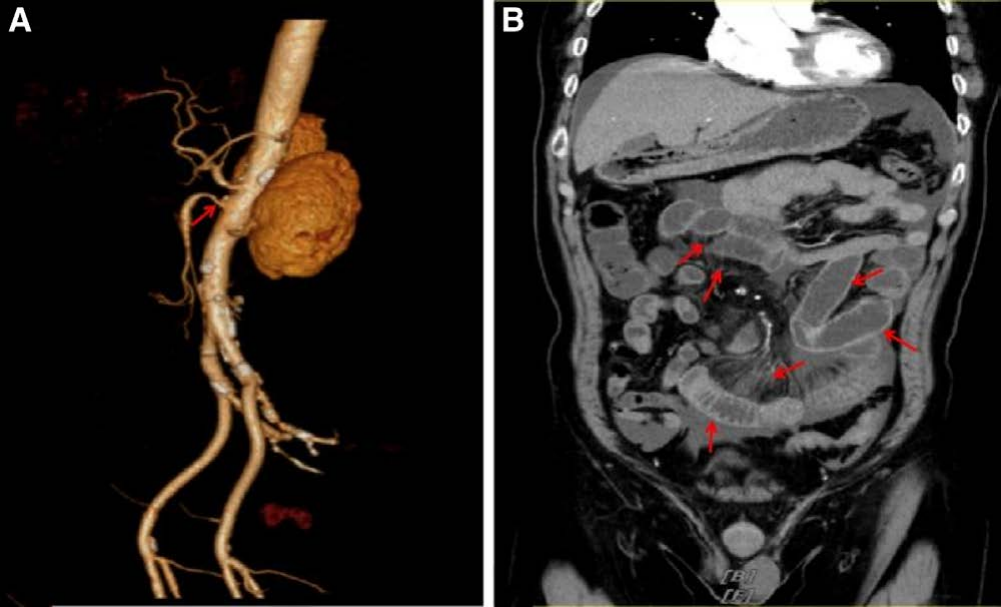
Abdominal CTA examination showing (A) stenosis at the opening of superior mesenteric artery and (B) ischemic changes in partial bowel and mesentery. CTA = computed tomography angiography.
